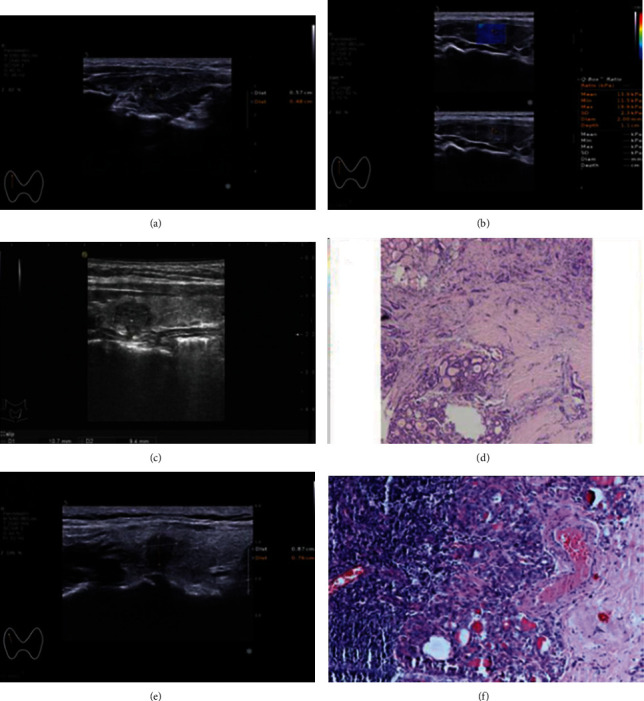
Ultrasonography of thyroid nodules. (a, b) Ultrasonographic appearance of a thyroid nodule pathologically diagnosed as a nodular thyroid goiter showing hypoechogenicity, unclear borders. (c, d) Ultrasonographic appearance of a thyroid nodule pathologically diagnosed as PTMC and negative for the BRAF V600E mutation, showing hypoechogenicity and central blood flow. (e, f) Ultrasonographic appearance of a thyroid nodule pathologically diagnosed with PTMC and positive for the BRAF V600E mutation, showing hypoechogenicity, unclear borders, and aspect ratios > 1.
